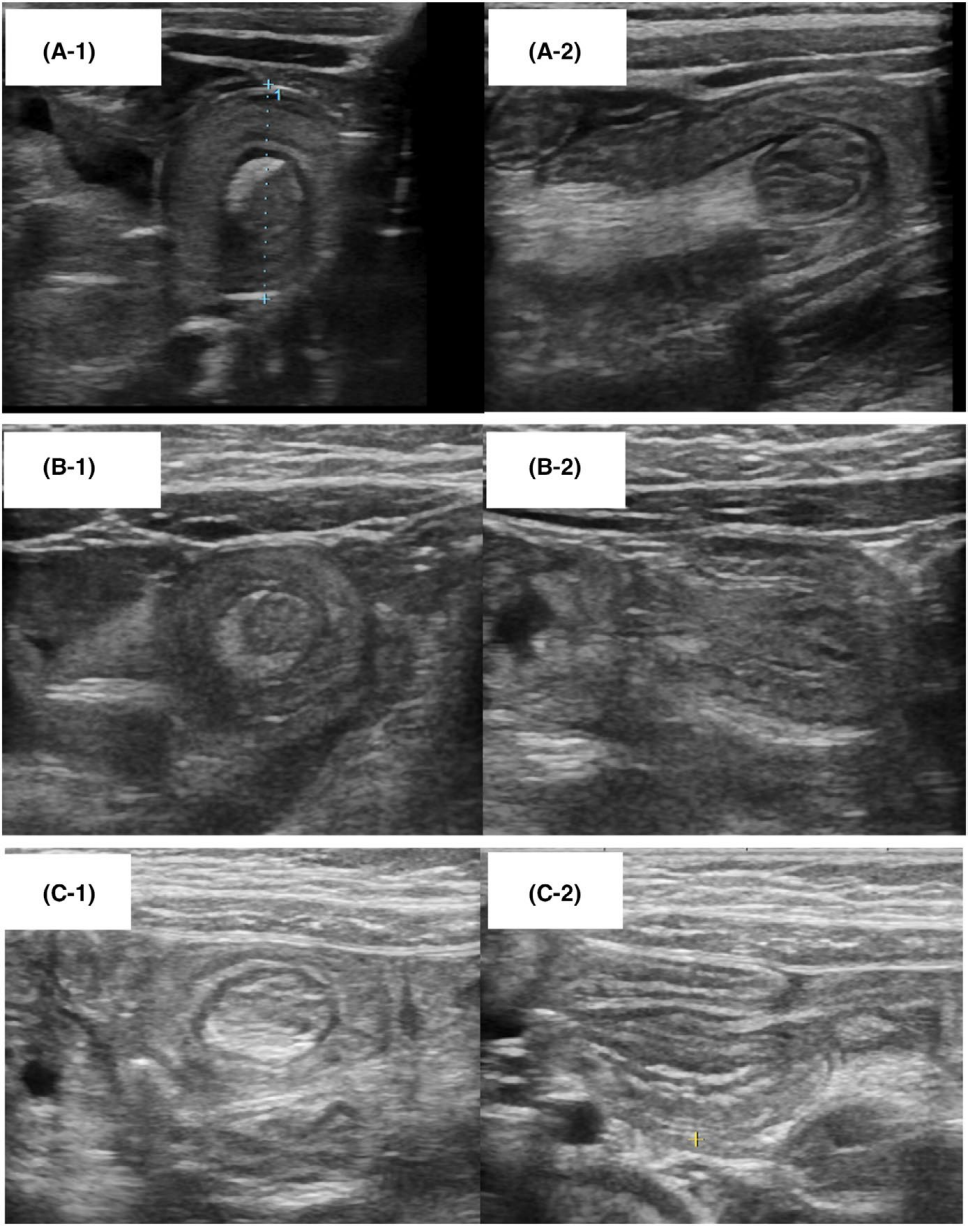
Ultrasound images of small intestinal intussusception in three OFC‐positive patients with FPIES. Observed on transverse scans as “donut signs” with multiple concentric rings, giving the target appearance, and on longitudinal scans as “sandwich sign” with a fork appearance. (A‐1) transverse scan of a 7‐month‐old boy; (A‐2) longitudinal scan of a 7‐month‐old boy; (B‐1) transverse scan of a 33‐month‐old girl; (B‐2) longitudinal scan of a 33‐month‐old girl; (C‐1) transverse scan of a 24‐month‐old girl; (C‐2) longitudinal scan of a 24‐month‐old girl.
